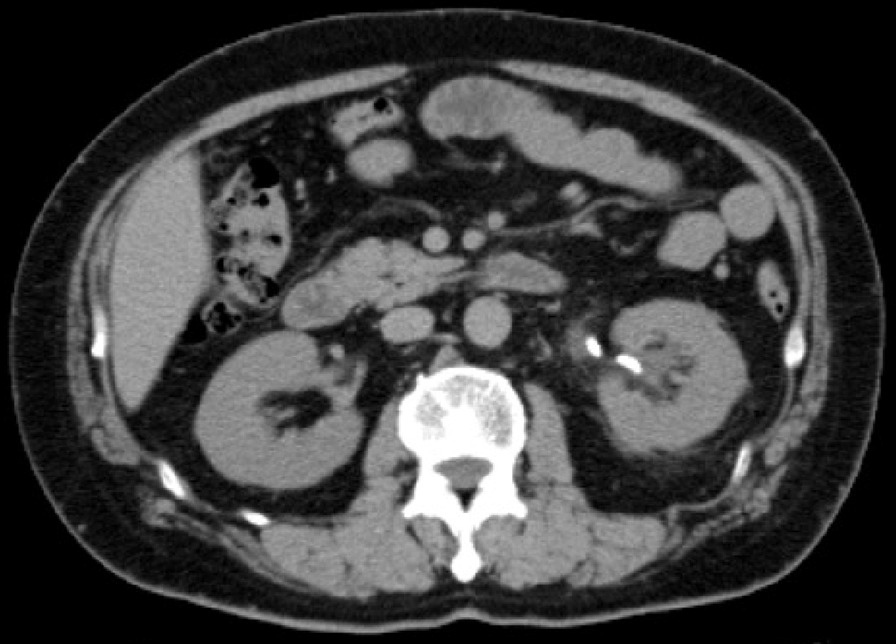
Plain thoracic, abdominal, and pelvic computed tomography. The white object in the left urinary tract is a double-J catheter, which was temporarily placed during transurethral ureterolithotripsy
